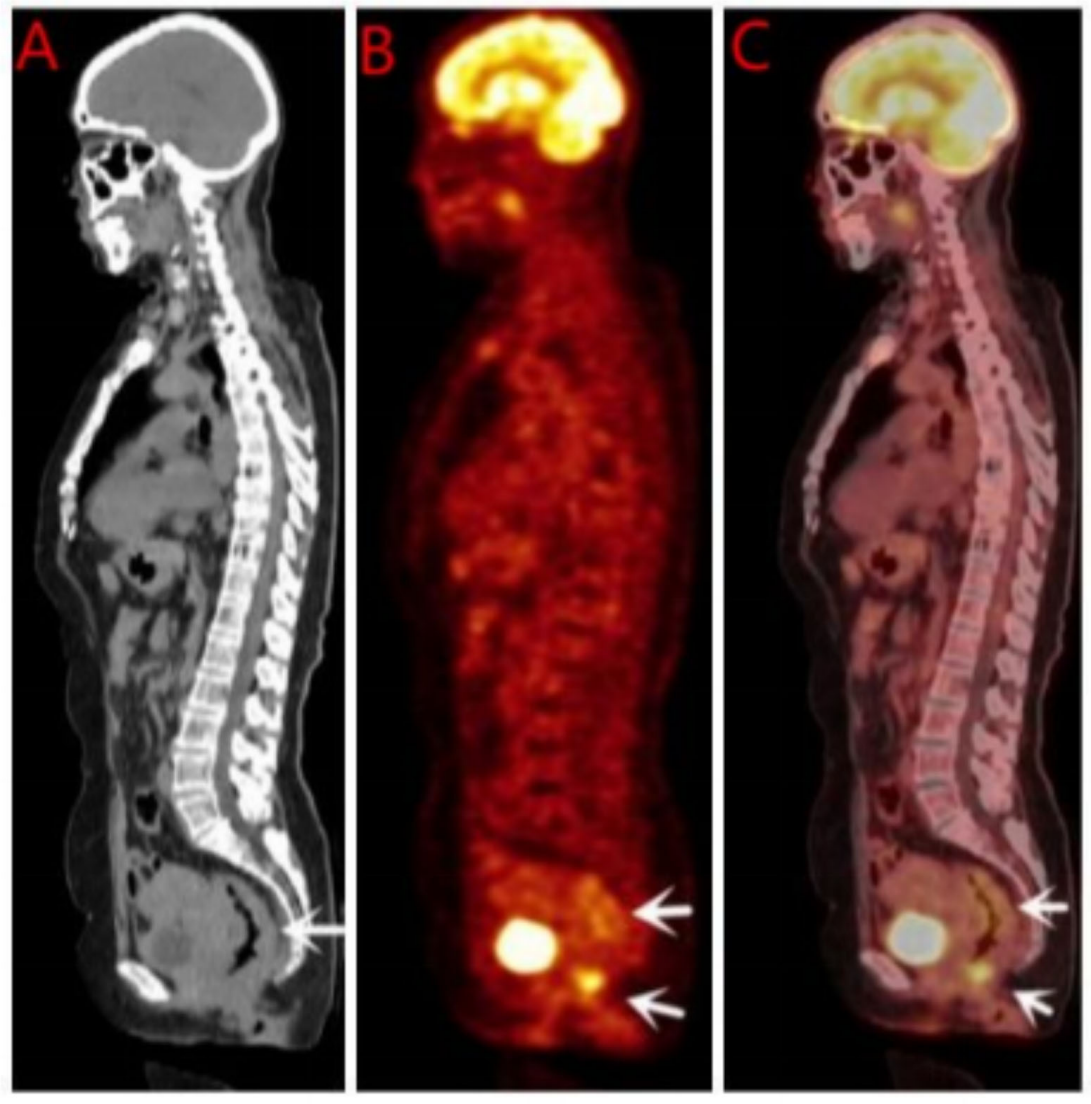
18F-FDG PET/CT before rectal treatment. (A) CT shows thickening of rectal wall; (B) PET shows high metabolism; (C) MIP shows of rectal wall. 18F-FDG PET/CT, 18F-flurodeoxyglucose positron emission tomography-computed tomography; MIP, Maximum Intensity Projection.
